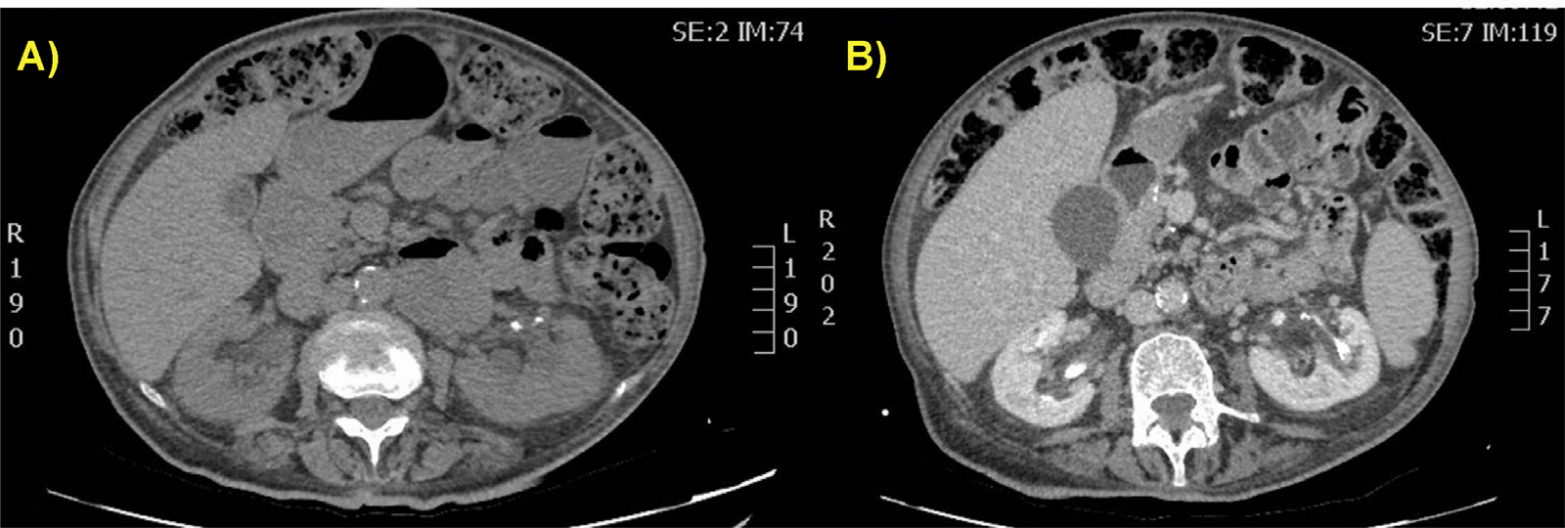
(A and B) Contrast-enhanced CT scan performed 6 months on the same patient before the bilateral renal hemorrhage occurred. Non-contrast (A) and venous (B) phases on axial planes showed regular morphology, dimension, and density of both kidneys in absence of expansive lesions. Left kidneys presented some calyceal calcifications.
